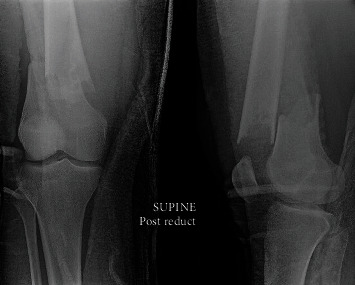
AP and lateral radiographs of the right knee demonstrating a pathologic fracture of the right distal femur. The lesion has characteristics consistent with nonossifying fibroma: lytic, cortically based lesion.
